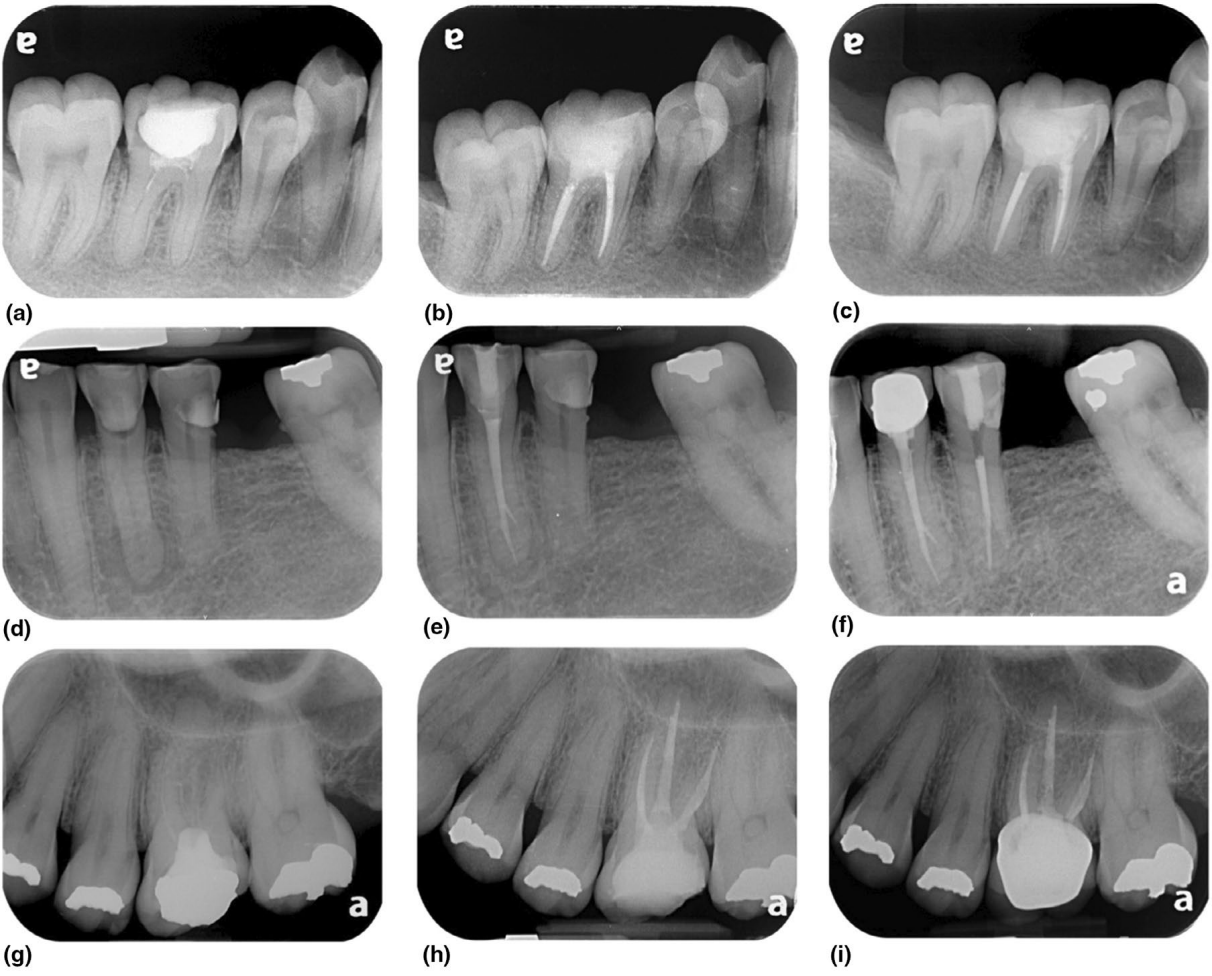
Representative outcome cases. (a–c) Retreatment case of a mandibular molar that healed after a 17‐month recall; (d–f) Mandibular first premolar that healed after a 12‐month recall; (g–i) Maxillary first molar with apical periodontitis after a 12‐month recall. Left column: Preoperative radiographs; Middle column: Postoperative radiographs; Right column: Recall radiographs.
